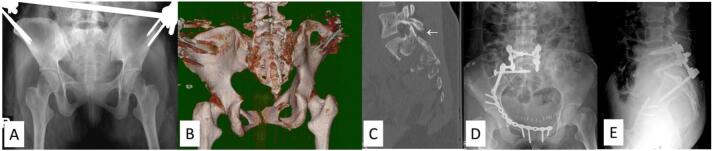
Demonstrating an AO type C1 sacral fracture, Isler subtype 3 (case No. 4). (A-C) Preoperative radiographs and CT reconstruction images showing right L5S1 joint subluxation with comminution and floating of the S1 superior facet (arrow). (D, E) Postoperative radiographs after unilateral lumbopelvic fixation, L5S1 posterior lumbar interbody fusion using a local chip graft, and a right iliac screw to reinforce the right iliosacral screw. Hardware removal was not performed in this case.
